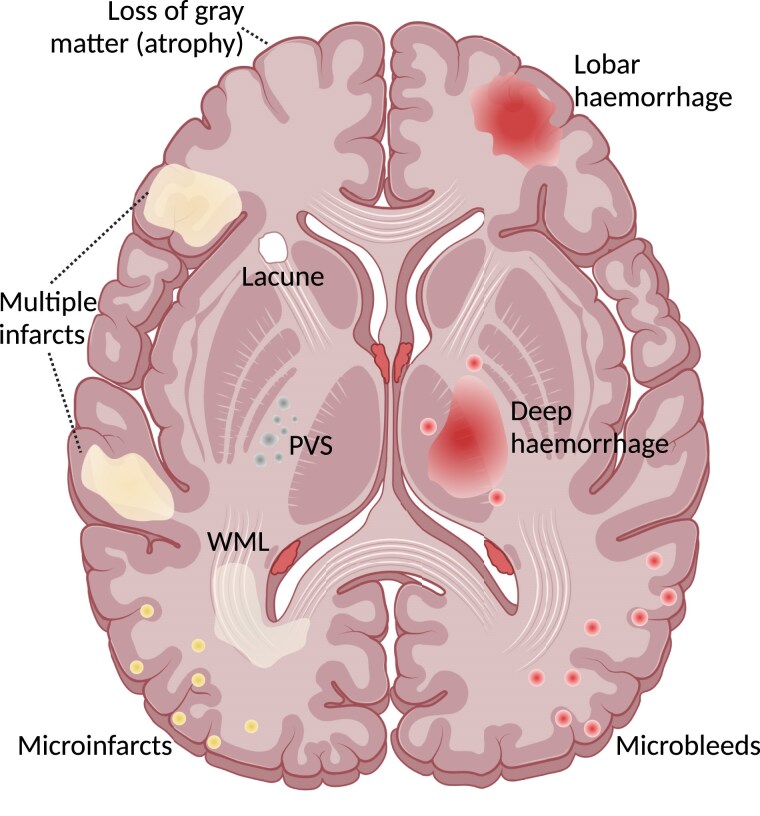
Manifestations of VaD. Horizontal section of the brain. WMLs show up as white matter hyperintensities on MRI. PVS, perivascular spaces; WML, white matter lesion.
